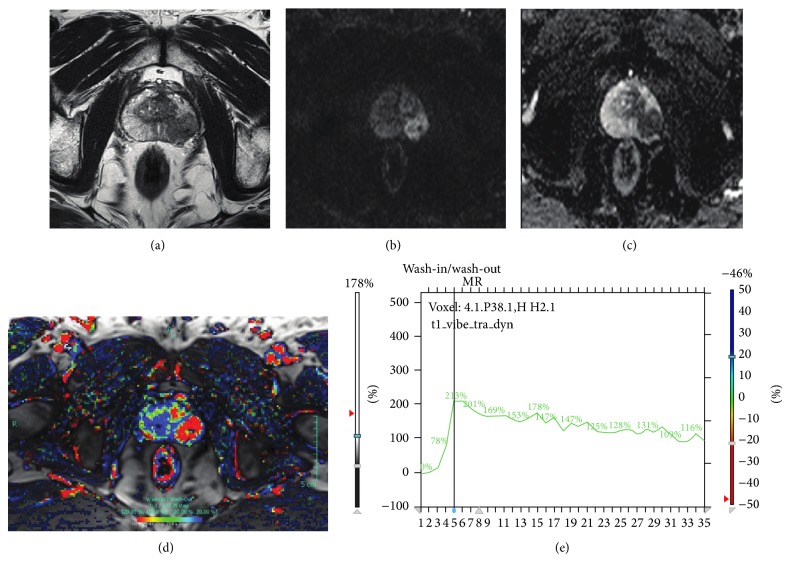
Multiparametric MRI data of a 67-years old male patient diagnosed with prostate cancer. T2w-MRI (a), DW-MRI (*b* = 1000) (b), ADC (c), DCE-MRI wash-in/wash-out map (d), and DCE-MRI time curve (e).
